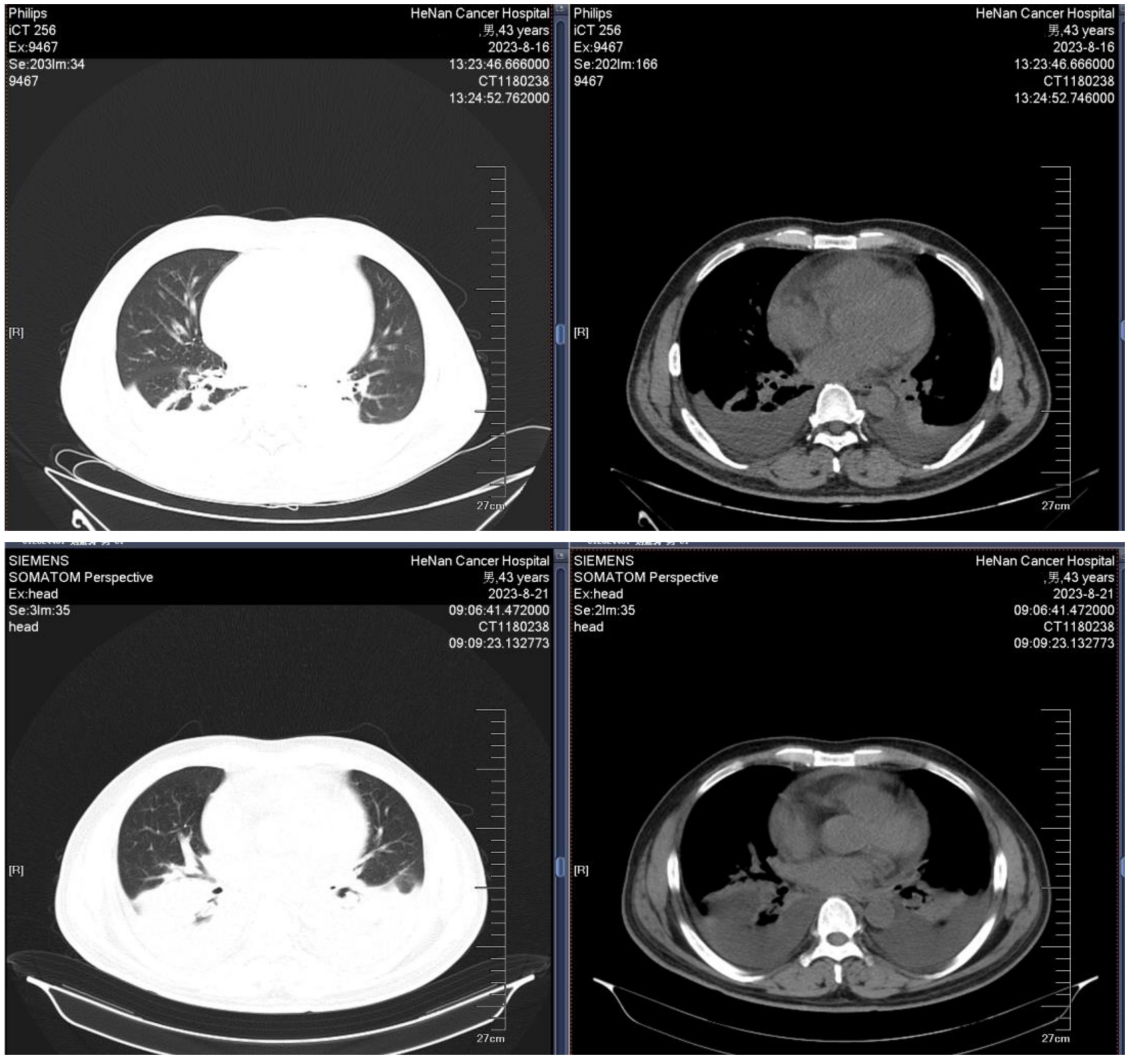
Example images of the chest CT during induction therapy in Patient 1.
